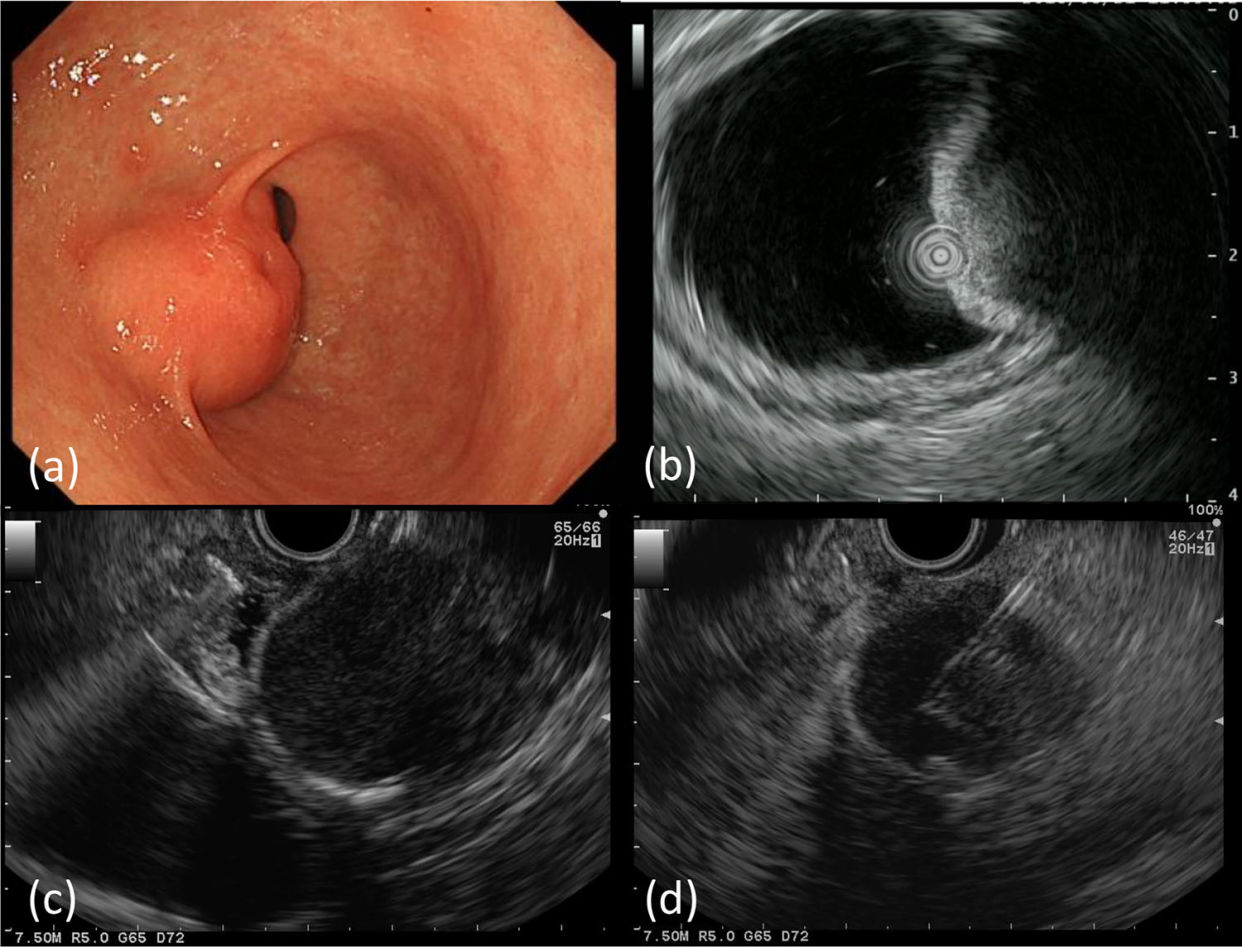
(a) Endoscopic examination shows the smooth elevated lesion with delle in the anterior wall of the gastric antrum. (b) Endoscopic ultrasound (EUS) shows a mass in the fourth layer of the gastric wall. (c) EUS shows a hypoechoic lesion that is 30 mm in diameter. (d) EUS images during EUS‐guided fine‐needle aspiration of the tumor.
